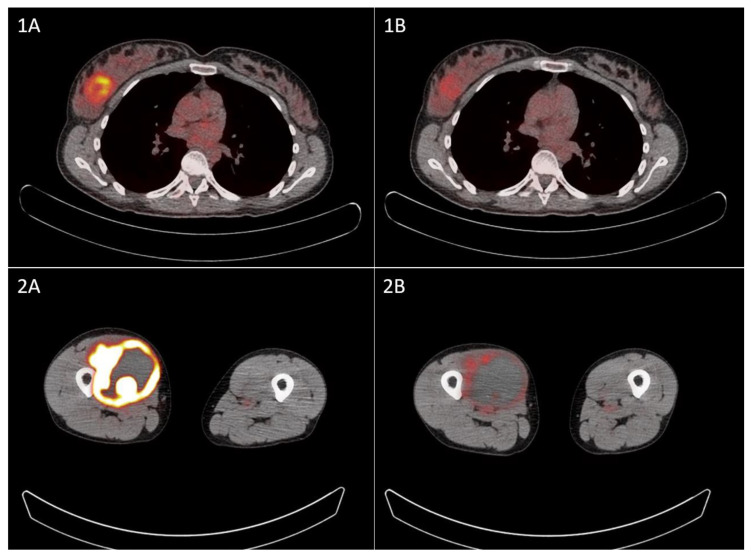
Baseline [^18^F]FDG PET/CT of an angiosarcoma of the breast (1A) and after one cycle of paclitaxel (1B), and the baseline [^18^F]FDG PET/CT of sarcoma NOS in the right upper leg (2A) and after 2 cycles of doxorubicine/ifosfamide (2B).
